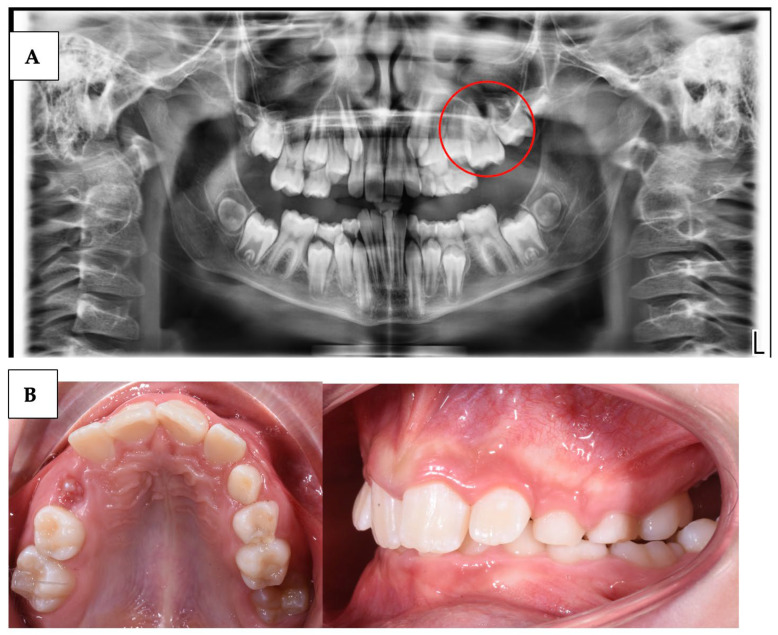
(A) Panoramic radiograph of a suspected case of PFE on tooth 26; (B) intraoral records of suspected PFE on tooth 26.
